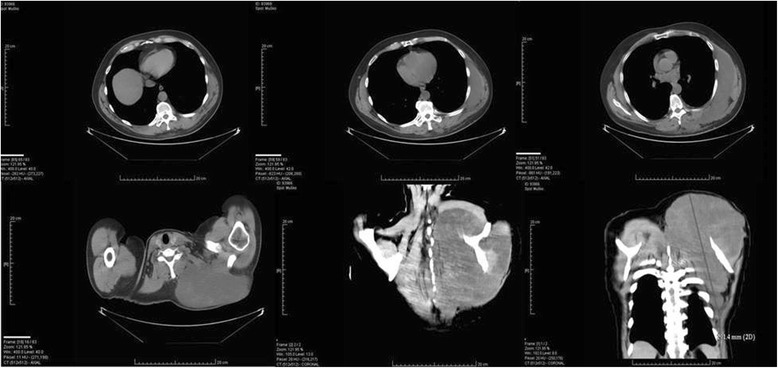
Computed tomography imaging of the chest showing giant tumor mass measuring 29 × 22 cm in size with destruction of the chest wall and propagation in to the chest cavity
